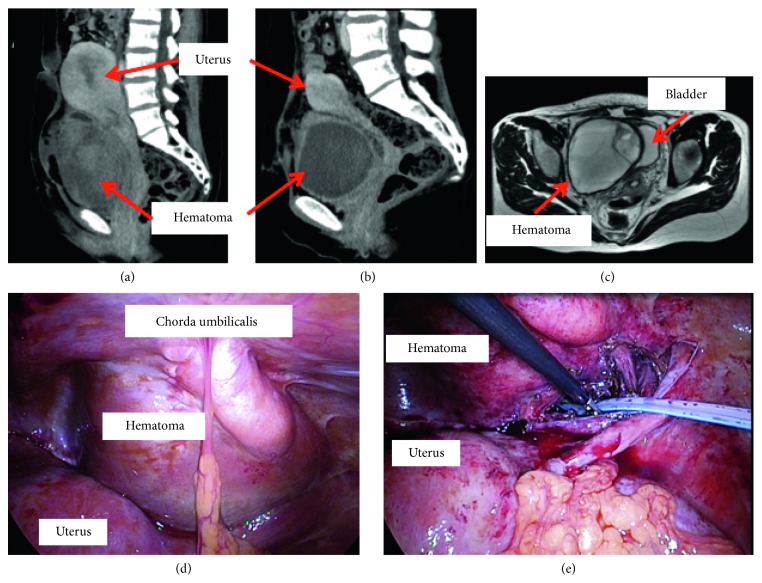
Occurrence of hematoma after caesarean section and laparoscopic surgery to treat it. (a) Contrast CT image taken on the 3rd postoperative day. Hematoma of 9 cm in diameter existed at the isthmus of uterus. Red arrow indicates hematoma and uterus. (b) Contrast CT image taken on the 28th postoperative day. Hematoma of same size remained in the same place. Red arrow indicates hematoma and uterus. (c) MRI (T2-weighted image) findings of hematoma taken on the 28th postoperative day. Encapsulated hematoma existed. Red arrow indicates hematoma and bladder. (d) Intraoperative findings. Hematoma existed extraperitoneally, inside chorda umbilicalis. (e) We cut Cutting of peritoneum to reach inside of hematoma. Drainage tube was set.
